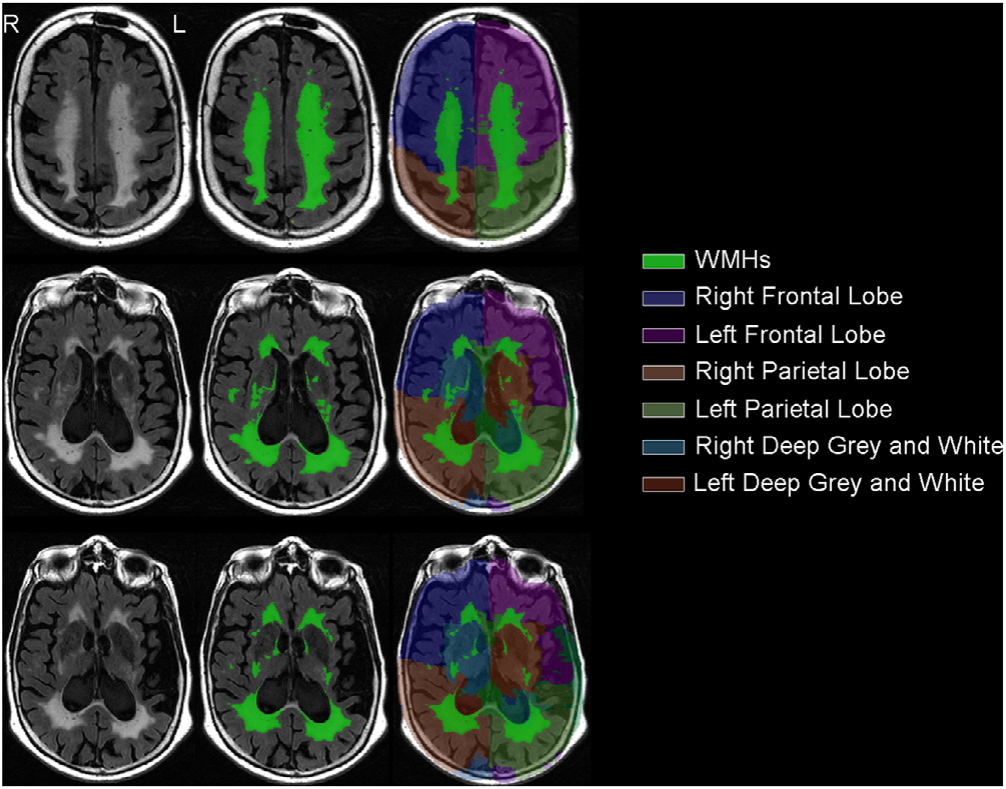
White matter hyperintensity identification and segmentation. Representative images showing 2D FLAIR MRI with superimposed WMH mask and WMH segmentation are shown. WMHs are identified in green, whereas the segmentation of the cortical lobes and deep gray and white matter of the basal ganglia are shown in the accompanying color atlas. 2D FLAIR, two‐dimensional fluid‐attenuated inversion recovery; MRI, magnetic resonance imaging; WMH, white matter hyperintensity.
